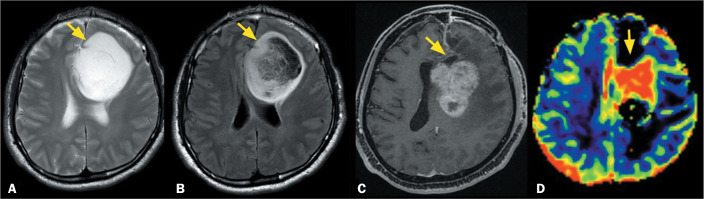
MRI scans of a 25-year-old male patient. A T2-weighted sequence (A)
